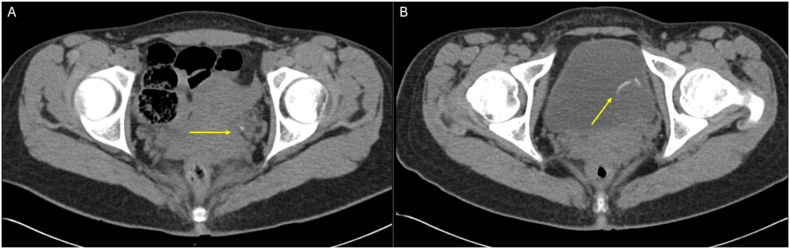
Legend: (A) Initial axial CT scan of abdomen and pelvis without contrast demonstrated moderate hydroureter tapering to a focal calcification in the distal ureter, originally interpreted as an obstructing calculus. (B) Follow-up CT scan without contrast at two months demonstrating progression of a heterogeneous mass. Note the presence of internal macroscopic fat and peripheral calcifications, suggestive of the osseous components within the botryoid rhabdomyosarcoma.
